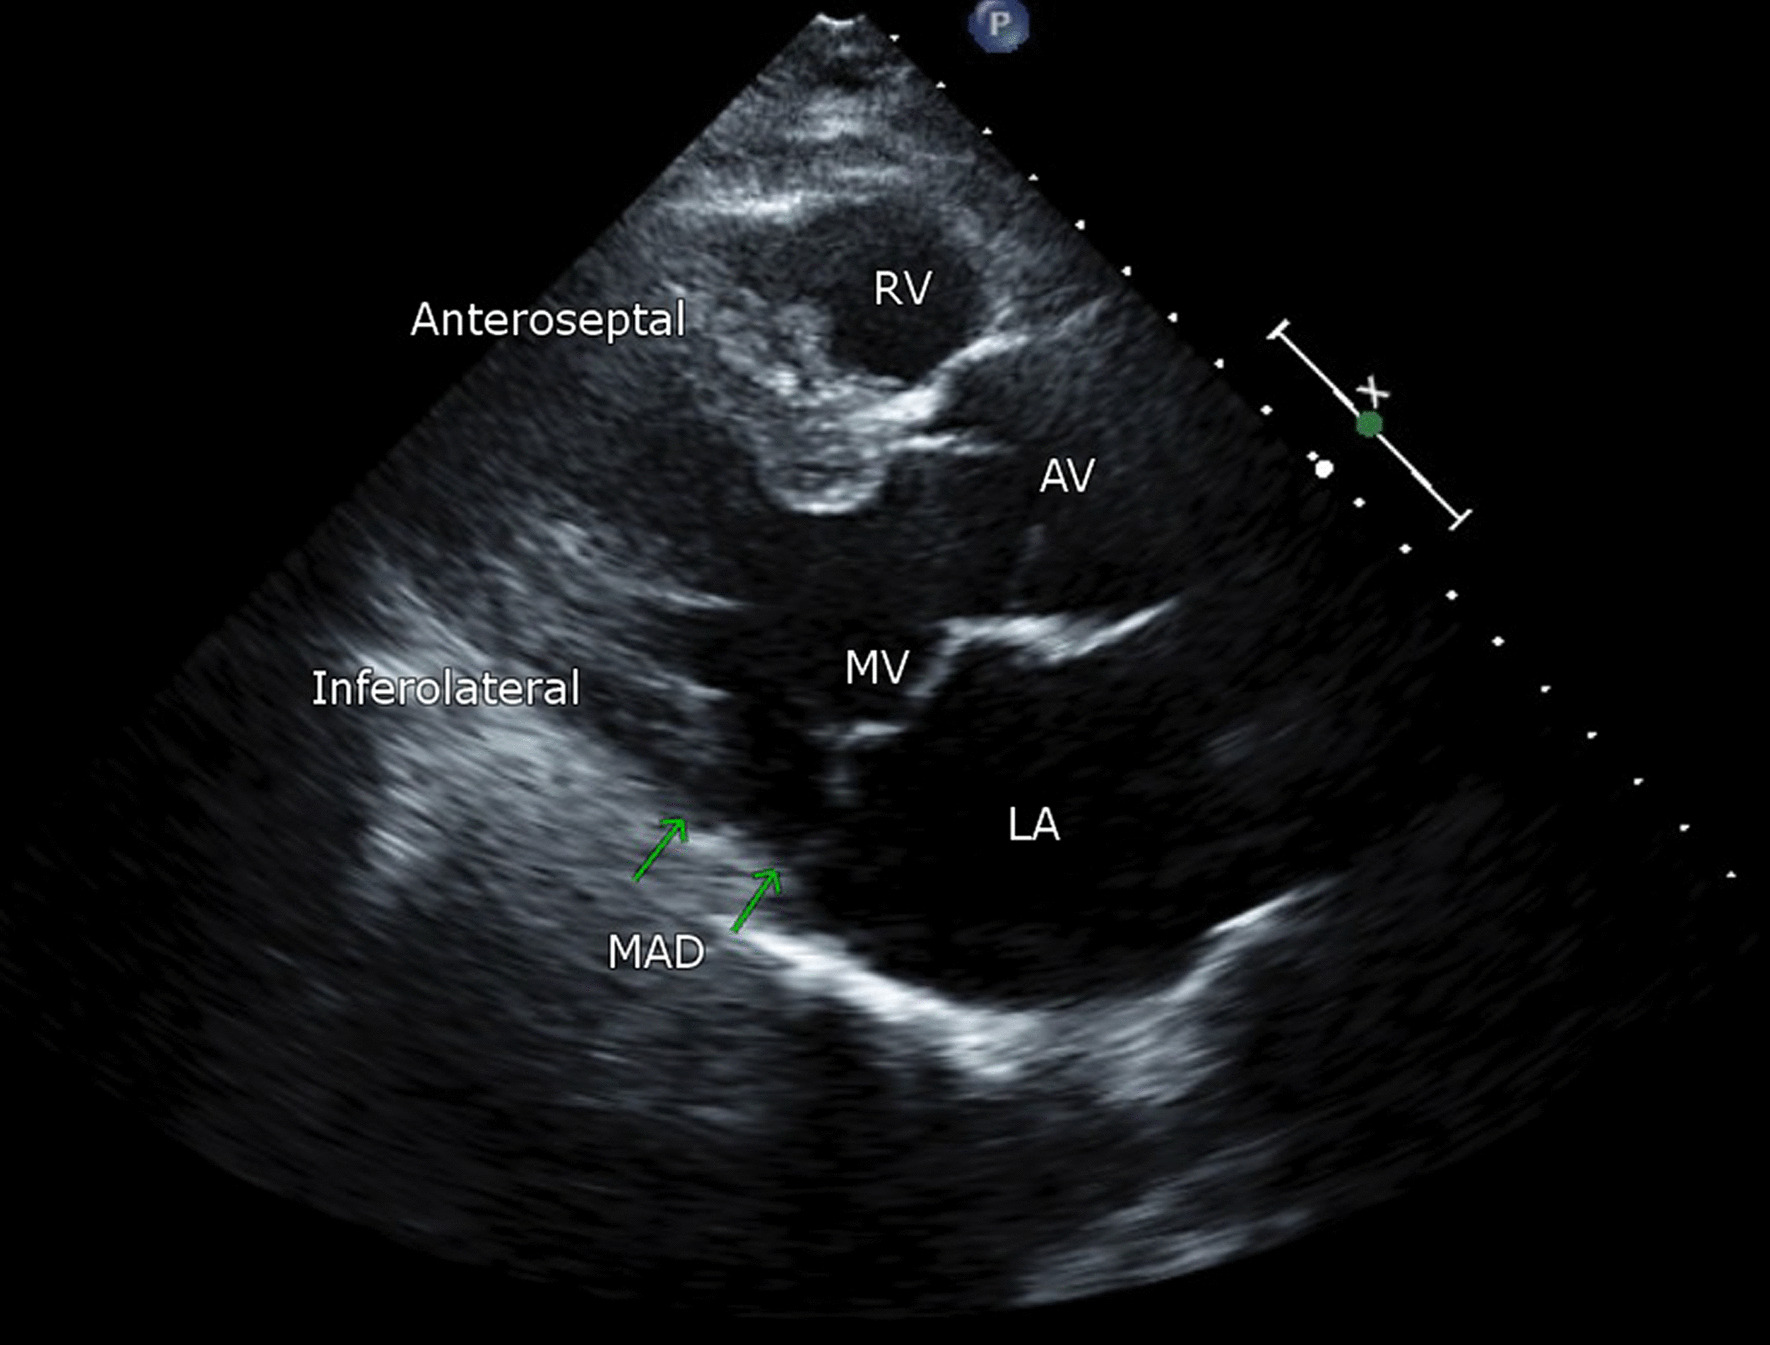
Parasternal long axis view at end systole demonstrating mitral annular disjunction. *RV* right ventricle, *AV*  aortic valve, *MV* mitral valve, *LA*  left atrium, *MAD* mitral annular disjunction
